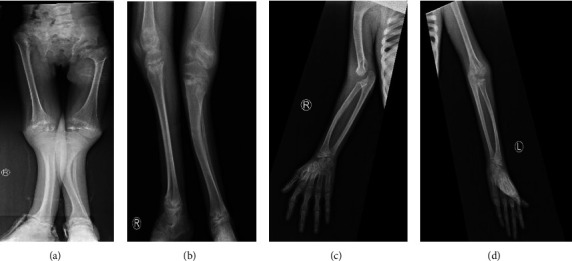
Radiographies. (A, B) Diffuse osteopenia and tubular bones are short. Metaphyseal sclerosis and irregularities more pronounced in and sclerotic femoral heads. Right hip dislocation with external the lower limbs. (C, D) Right humerus bowing deformity. Small, fragmented rotation of the right lower limb. Bilateral coxa varus. Left genu valgus. Bowing deformity of the left tibia. Metacarpals and metatarsal are short.
